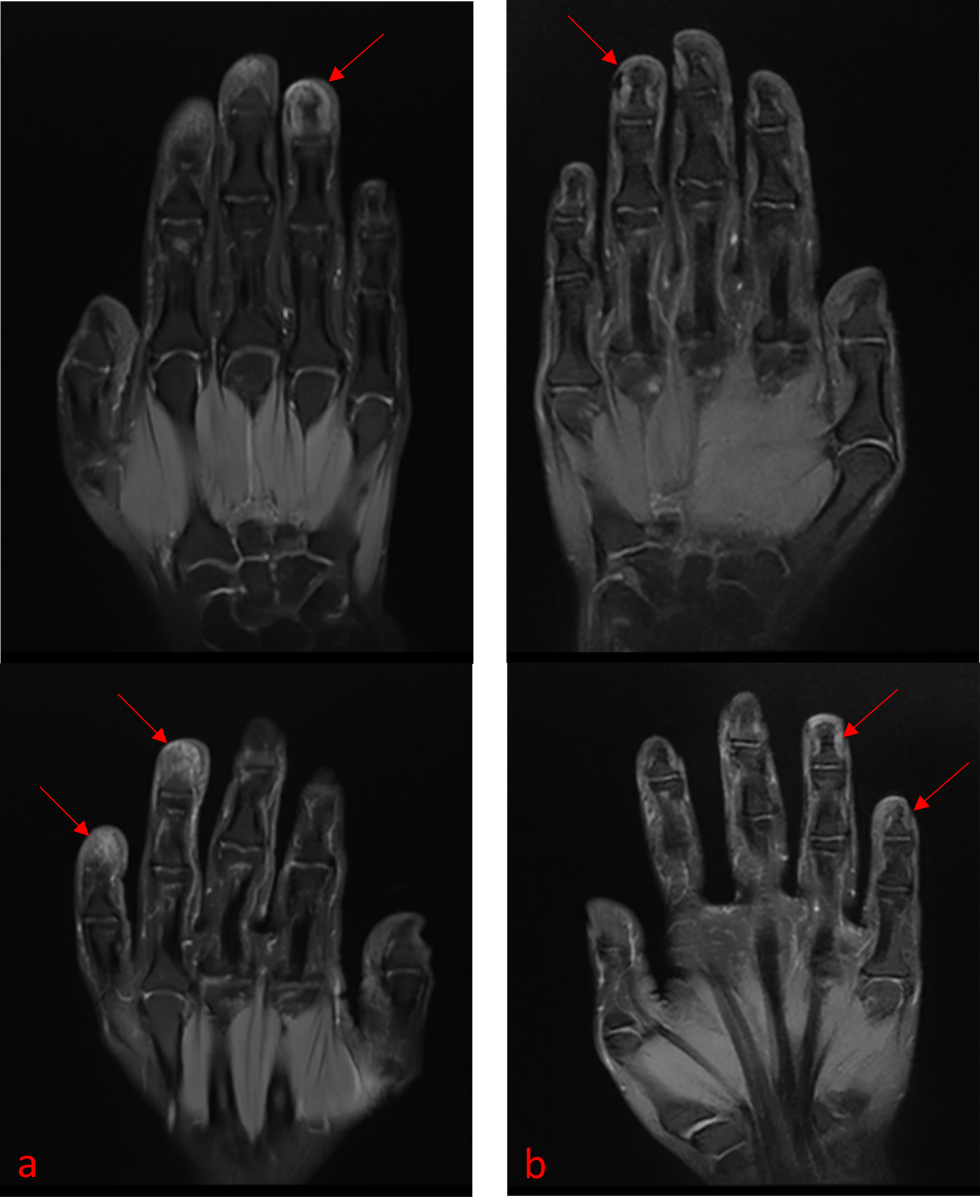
MRI findings at baseline and after treatment with Xeligekimab. The post-treatment COR T2WI-FS sequence indicated marked improvement and partial resolution of the bone-marrow edema in the distal phalanges of the left fourth and right fourth and fifth fingers.
